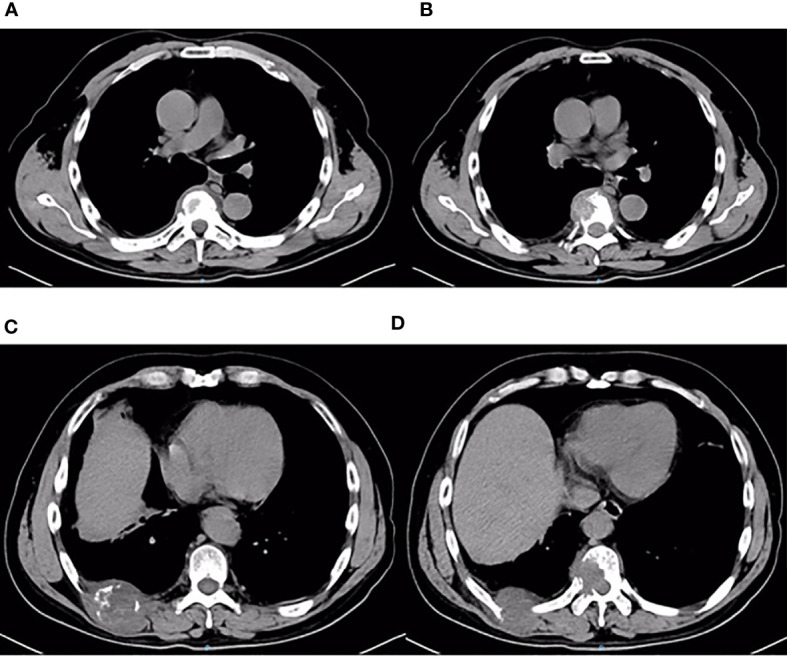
Thoracic and abdominal CT findings on December 6, 2019. Thoracic and abdominal CT revealed multiple metastases of the chest wall and thoracic vertebrae (A–D). Metastases of the third anterior ribs on the left, T7 vertebrae and its appendages, ninth posterior ribs on the right, and T10 vertebrae and its appendages.
